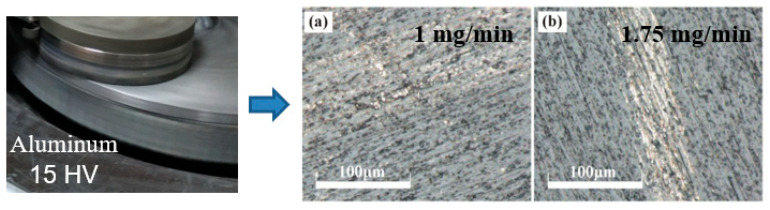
Optical images after lapping using aluminum disc: (a) common lapping; (b) ultrasonic-assisted lapping.
